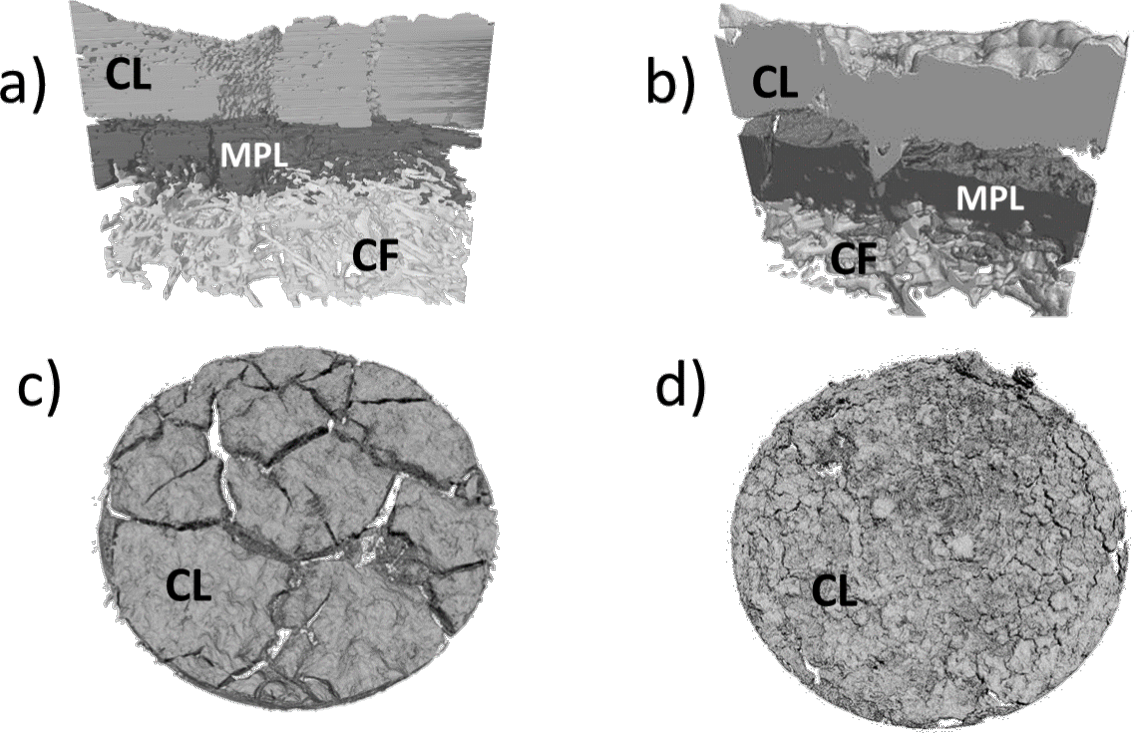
3D rendering of the X-ray microtomography data for the electrode cross section (a) coated electrode (b) sprayed electrode. 3D rendering of the X-ray microtomography data for the electrode surfaces (c) coated electrode (d) sprayed electrode. CL, MPL, and CF stand for catalyst layer, microporous layer, and carbon felt, respectively. Reprinted with permission from [12]. Copyright 2014 Elsevier.
